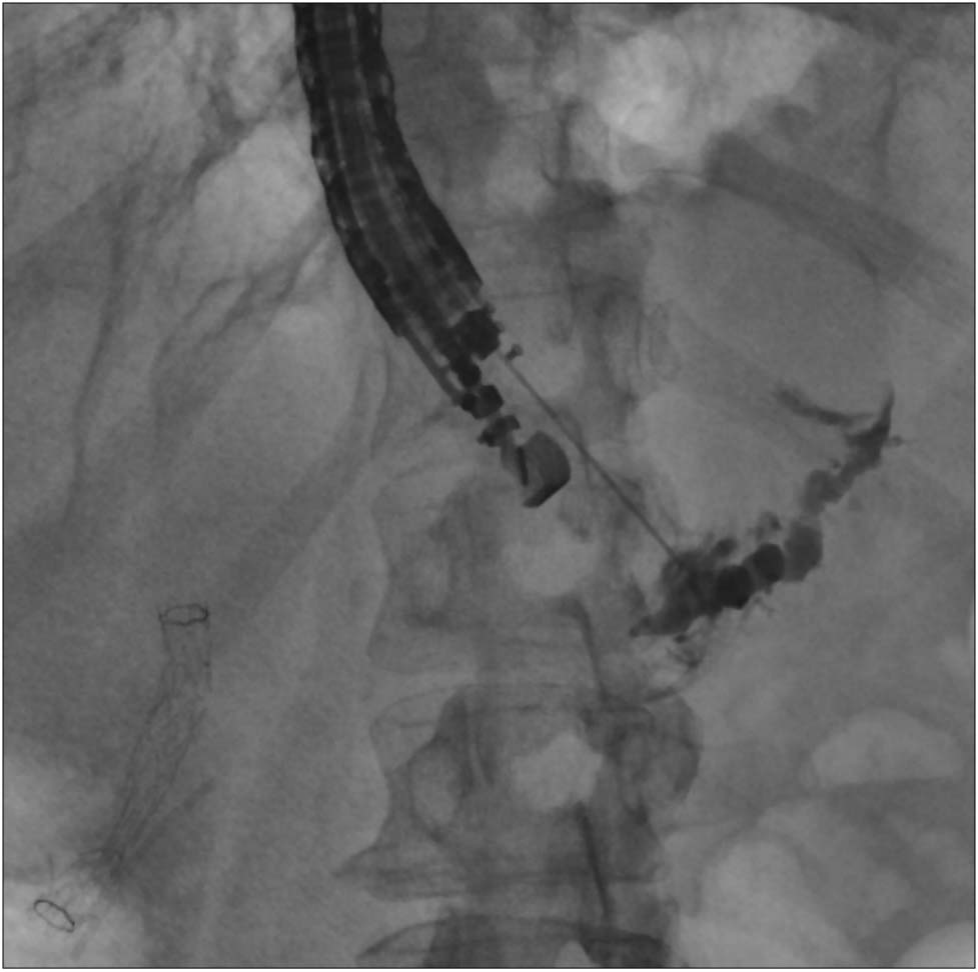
Endoscopic ultrasound-guided pancreaticogastrostomy was created distal to the stricture. Pancreatogram confirms area of contrast extravasation and dilated pancreatic duct in the distal body and tail of pancreas.
